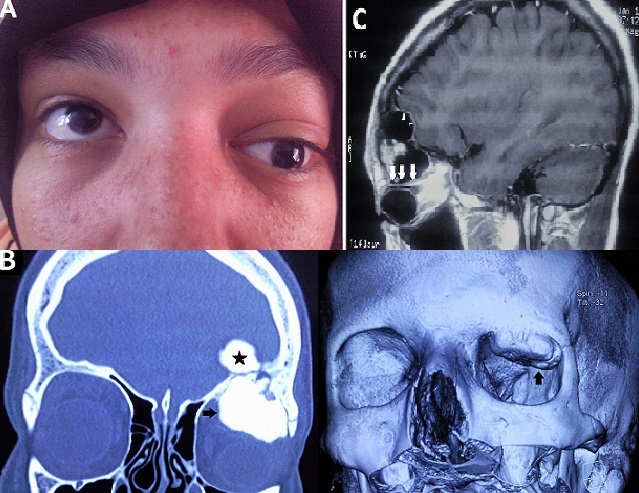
A): proptosis of the left eye with total ophthalmoplegia; B): coronal computed tomography (CT) and Three dimensional CT image of the cranium showing a right hyperdense intraorbital mass of the horizontal part of the frontal bone arising from its lateral side (black arrows) with an extension to the endocranium (star) C): Magnetic Resonance Imaging showing anterior displacement and compression of the left eyeball (white arrows) and the frontal lobe (white arrowheads)
